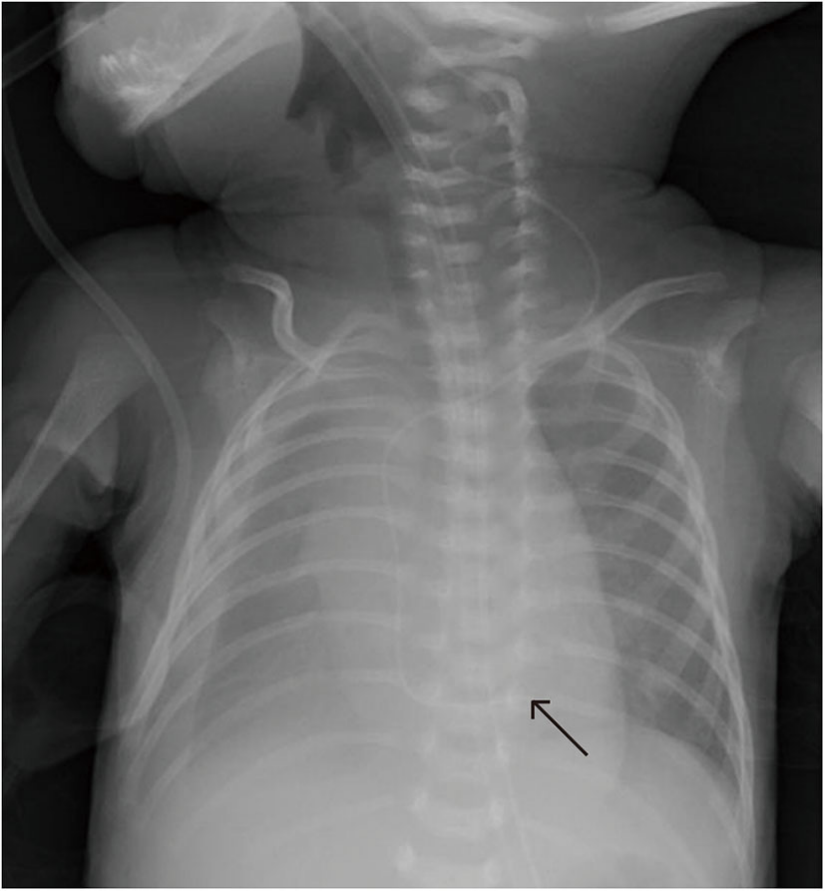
Patient E underwent uneventful Epicutaneo cava catheter (ECC) insertion, but several hours after ECC infusion, the routine postoperative chest X-ray showed that the tip was too deep.
